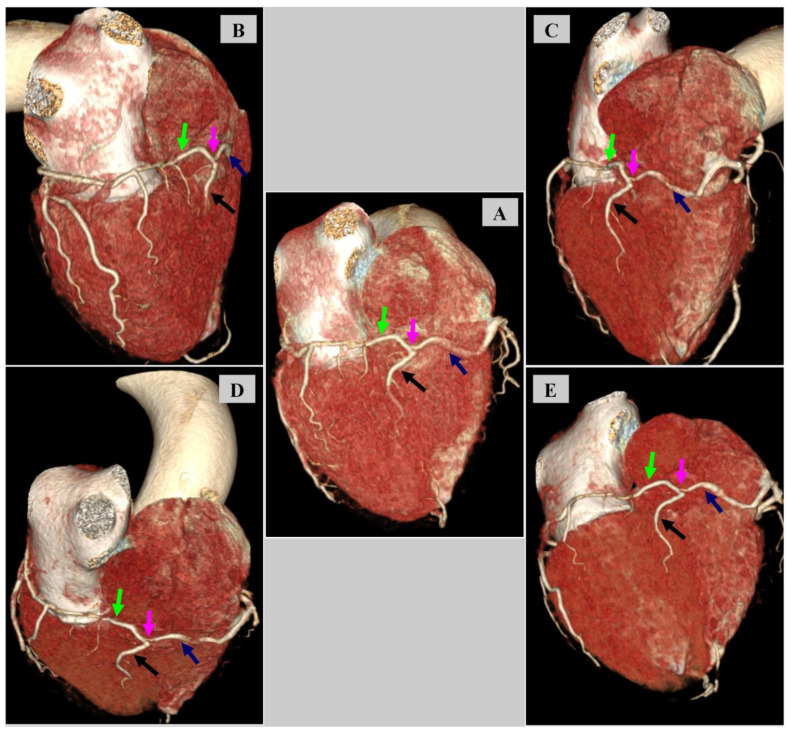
Coronary computed tomography angiography. Angiographic phase. 3D volume-rendering technique (VRT). The anomalous artery of Kugel. The green arrow indicates the left circumflex (LCx), the blue arrow indicates the right coronary artery (RCA), and the black arrow indicates the posterior descending artery (PDA). The magenta arrow indicates the Kugel artery. (A) Oblique view focused on the artery of Kugel. (B) Oblique view of the artery of Kugel from the LCx side. (C) Oblique view of the artery of Kugel from the RCA side. (D) Oblique view of the artery of Kugel from the atria side. (E) Oblique view of the artery of Kugel from the apical side.
